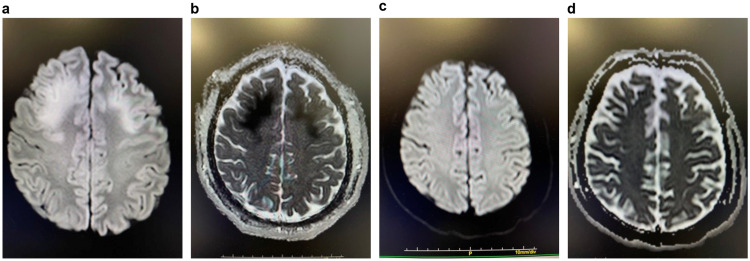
Magnetic resonance imaging findings on day eight. A. Brain MRI on day eight of hospitalization: diffused-weighted image showing abnormal signals from the cortex to the subcortical region of the bilateral frontal lobe apex. B. Brain MRI on day eight of hospitalization: the apparent diffusion coefficient map is low. C. Brain MRI on day 28 of hospitalization: previously noted abnormal signals from the cortex to the subcortical region of the bilateral frontal lobe apex have disappeared. D. Brain MRI on day 28 of hospitalization: the low signal of the apparent diffusion coefficient map has disappeared. MRI, magnetic resonance imaging.
